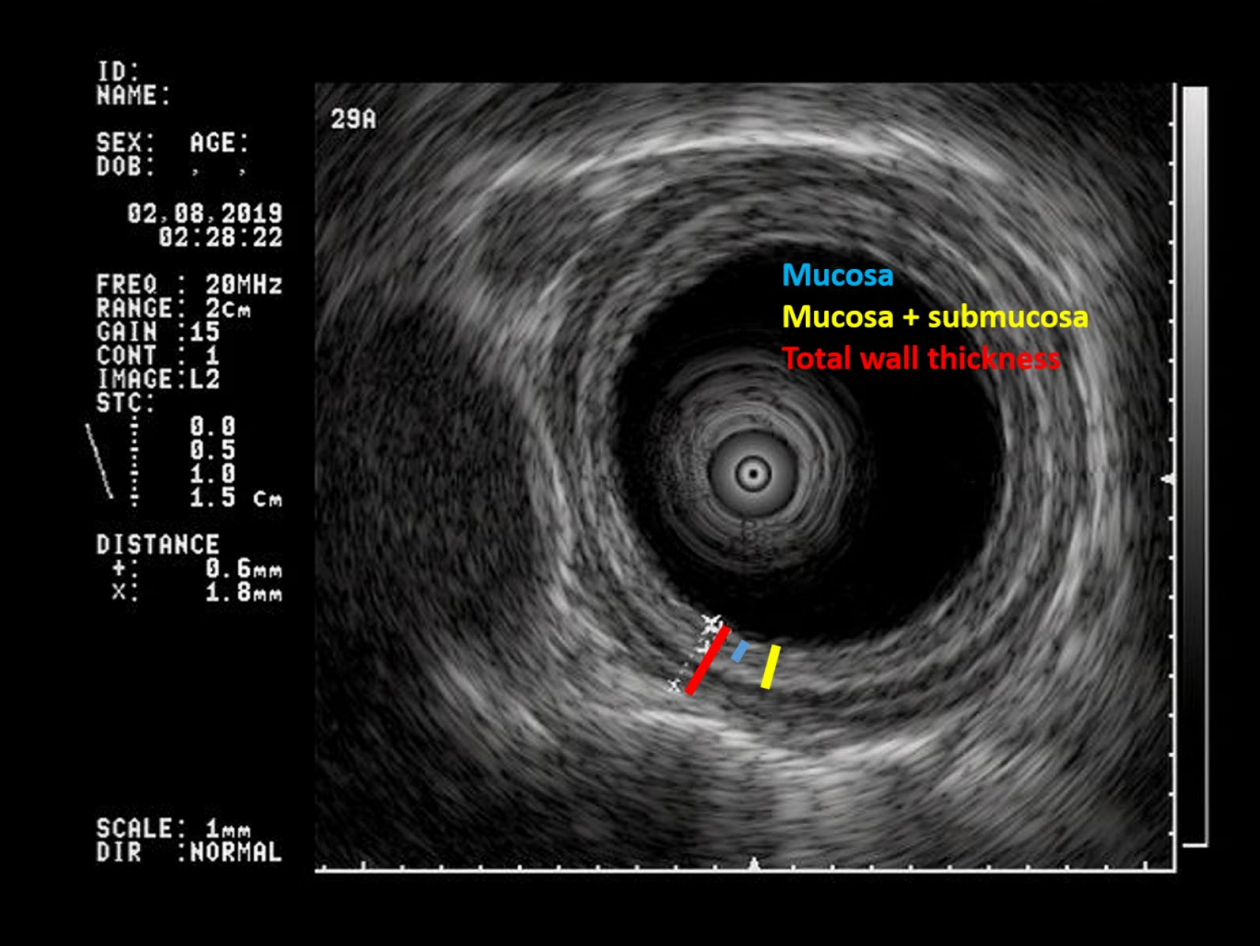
Endoscopic ultrasound images obtained using a miniprobe inside of a water filled latex balloon sheath to provide acoustic coupling in an 11-year-old boy. The blue bar defines the mucosa. The yellow bar defines the mucosa and submucosa. The red bar defines the full thickness of the esophageal wall. In our protocol, the muscle layer is derived by subtracting the yellow bar (mucosa plus submucosa) measurement from the red bar, the full esophageal wall thickness. In the figure, the thin hyperechoic band in the muscularis (beyond the yellow bar but within the red bar) represents the border between the circular and longitudinal muscles
